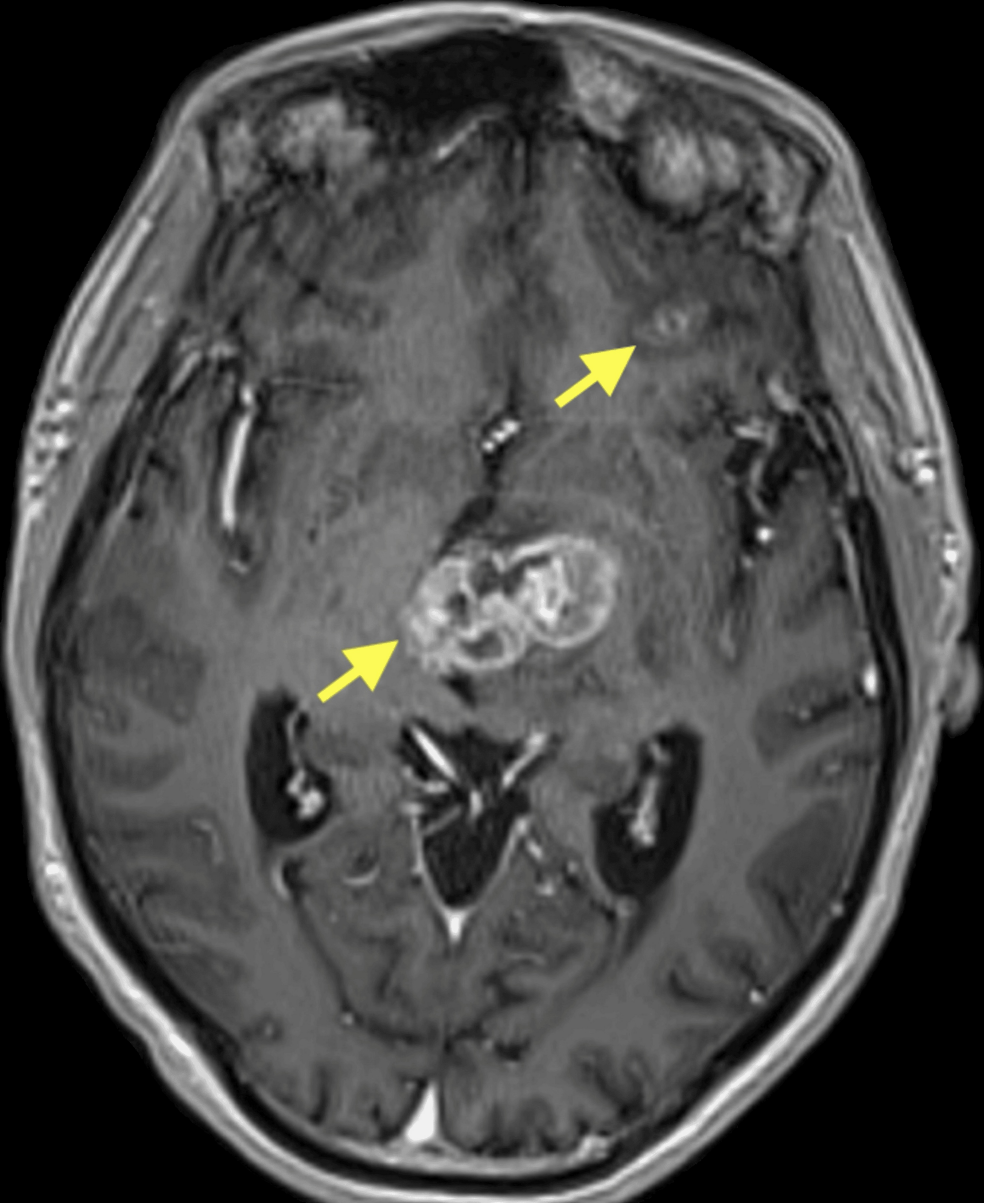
MRI of the brain T1 with contrast. MRI of the brain demonstrating the ring-enhancing multiple lesions in the left thalamus and the left frontal lobe (yellow arrows).
